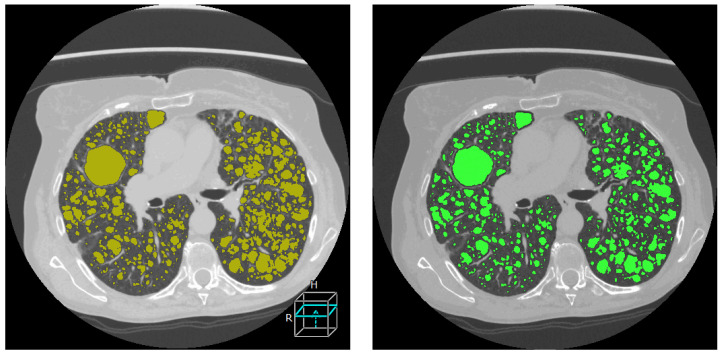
An example of the standard semi-automatic cyst segmentation (left) and fully automatic cyst segmentation (right) of an axial section of the chest CT scan of a patient with LAM. The cystic areas are highlighted in chartreuse color in the image on the left, and green color in the image on the right.
